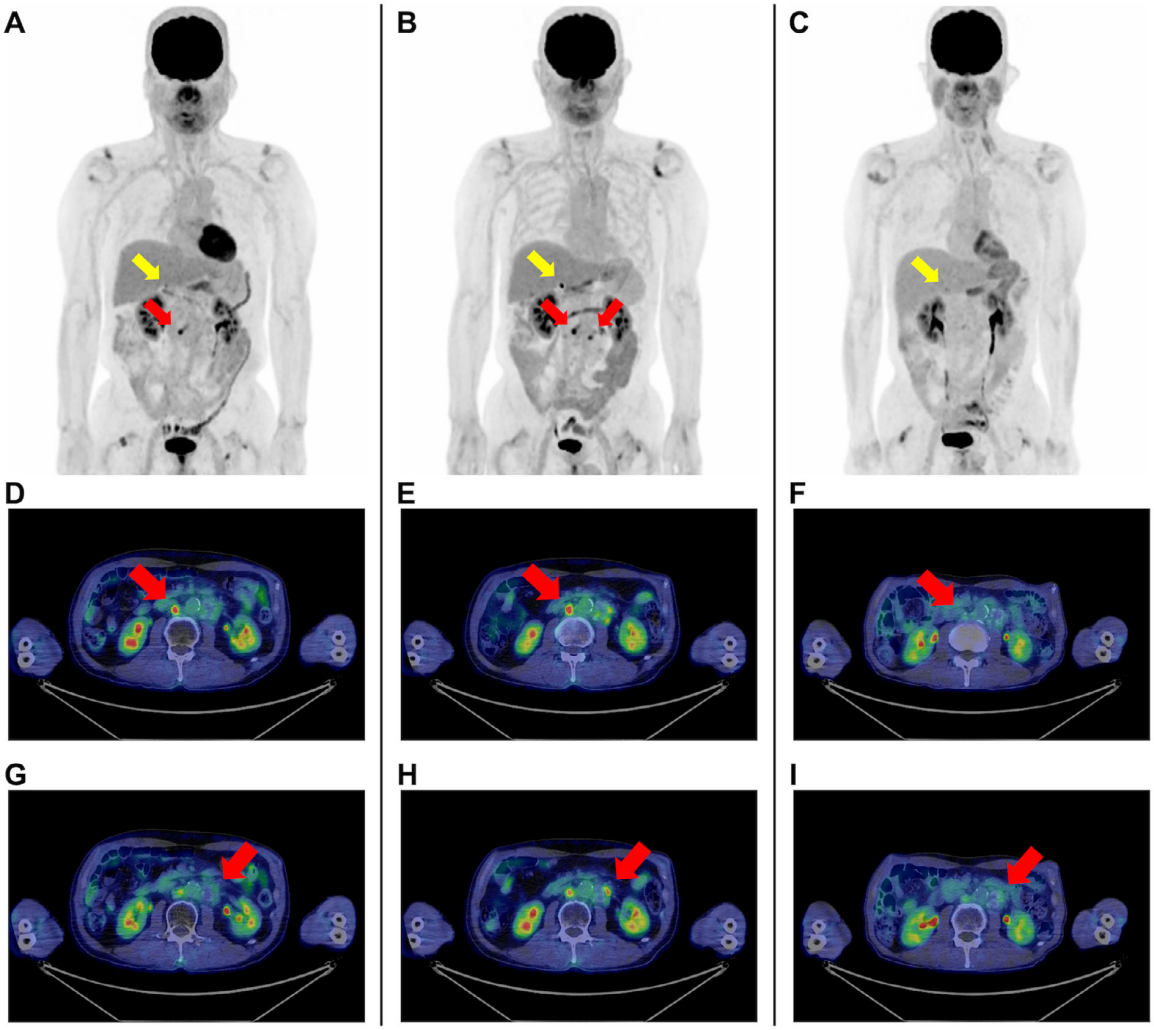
Positron emission tomography–computed tomography (PET–CT) during clinical course. (A–C) Whole‐body maximum intensity projection (MIP) images. (D–I) Axial PET–CT images of the right (D–F) and left (G–I) para‐aortic lymph nodes. Red arrows indicate the para‐aortic lymph nodes of interest, and yellow arrows indicate the right adrenal gland. (A, D, G) After two cycles of epcoritamab (pre‐allogeneic haematopoietic stem cell transplantation [allo‐HSCT]), residual fluorodeoxyglucose (FDG) uptake was observed in the right para‐aortic lymph node (D, maximum standardized uptake value [SUVmax] 7.9), with no significant uptake in the left (G, SUVmax 2.5). Mild physiologic uptake was noted in the right adrenal gland (SUVmax 4.2). (B, E, H) On Day +49 post‐allo‐HSCT, persistent FDG uptake was observed in the right para‐aortic lymph node (E, SUVmax 8.1), with newly appearing uptake in the left (H, SUVmax 5.0) and in the right adrenal gland (SUVmax 11.4), indicating progressive disease. (C, F, I) After nine cycles of epcoritamab post‐allo‐HSCT relapse, complete metabolic response (F, SUVmax 3.0; I, SUVmax 2.4). Mild physiologic uptake remained in the right adrenal gland (SUVmax 3.9).
